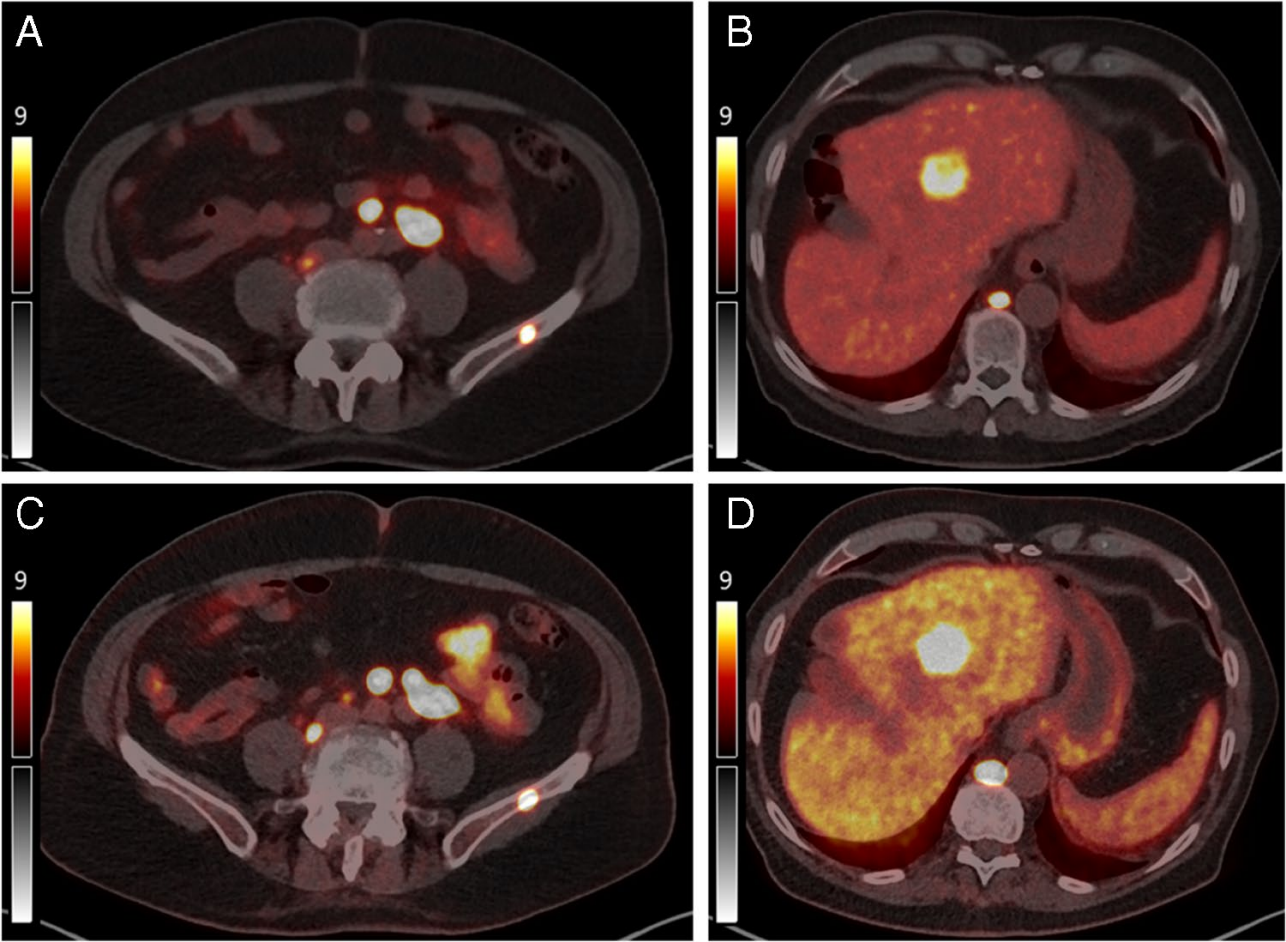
Example images for patient without mismatch lesions. A and B show images post-[^68^ Ga]Ga-PSMA-11 PET/T and C and D post-additional PET/CT with FDG. All lesions (lymph nodes, bone, and liver) demonstrate intense uptake in both scans. No new lesions are visible at additional imaging with FDG
